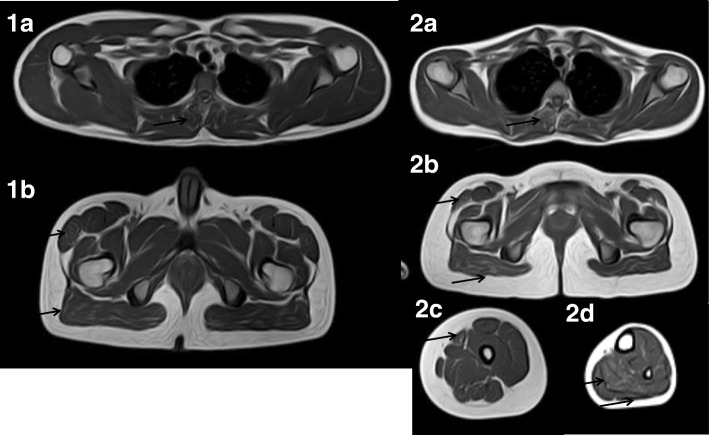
Whole-body magnetic resonance imaging T1-weighted axial sections in both siblings (1a and 1b: boy; 2a–2d: girl). Involved muscles are depicted by *black arrows*. 1a and 2a: Mild hypersignal of thoracic extensors. 1b and 2b: Mild hypersignal of tensor fasciae latae and gluteus maximus. 2c: Sartorius mild hypersignal. 2d: Soleus mild hypersignal and medial gastrocnemius atrophy
